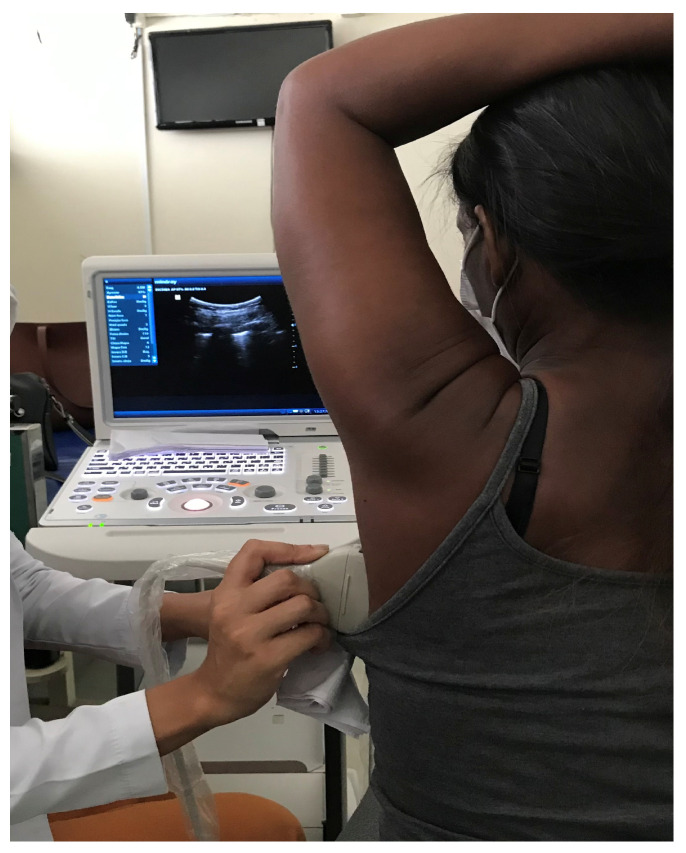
Acquisition of lung ultrasound images in a patient diagnosed with SSc-ILD. Source: author.
